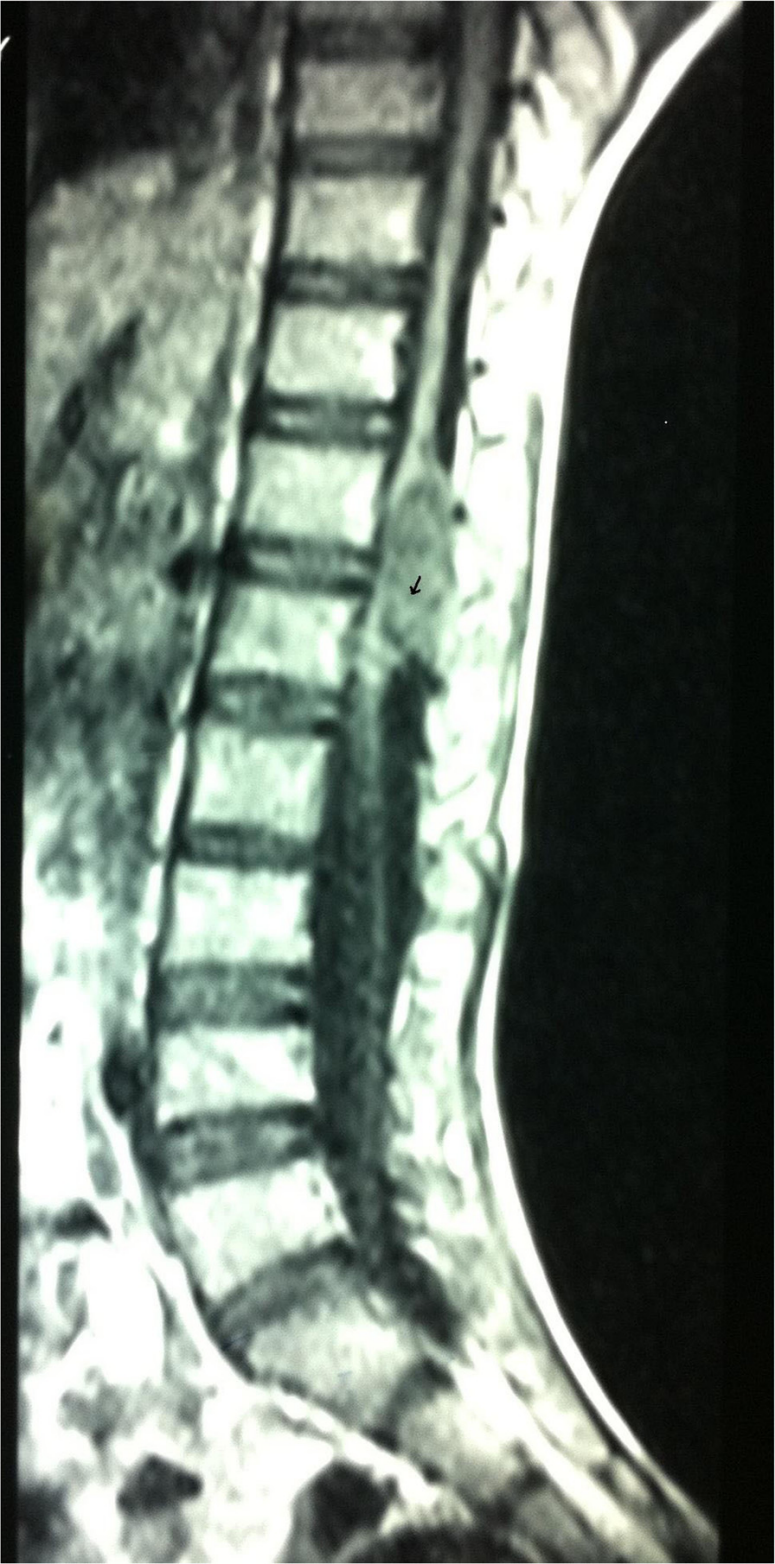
Sagittal T1-weighted image shows a hypointense lesion with a hyperintense signal area at the lower end (arrowhead).
